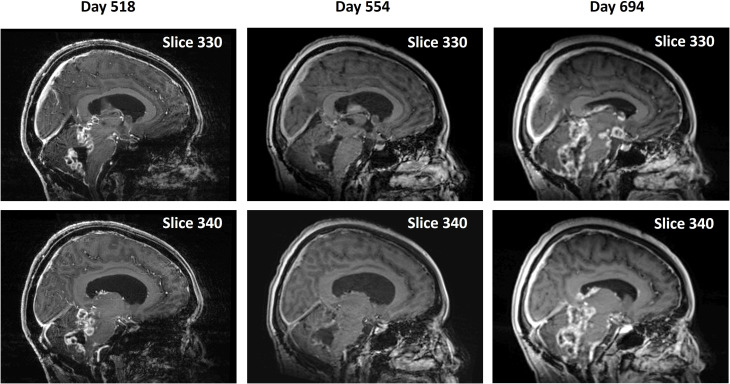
Second recurrence and remission of tumor during continued OMT and subsequent progression. Pairs of midline sagittal parasagittal T1-weighted post-contrast MRI scan slices during continued OMT showing second recurrence of the tumor on Day 518, its remission following OMT and radiation treatment on Day 554 and third recurrence on Day 694.
